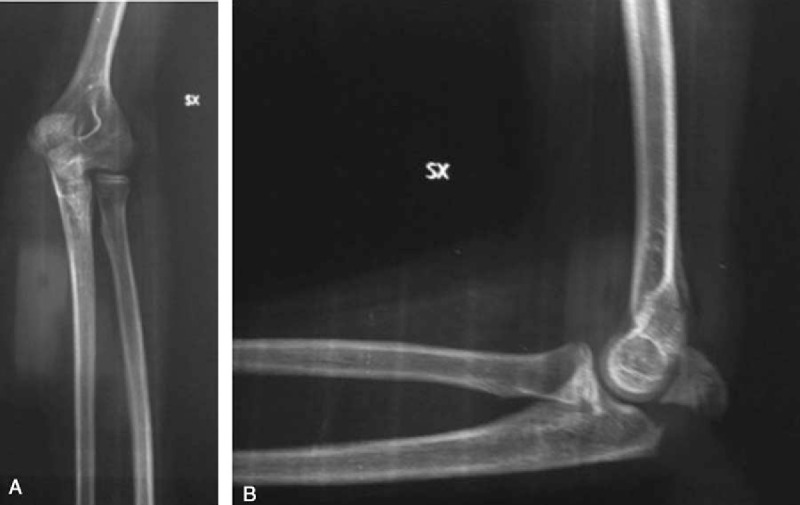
Preoperative X-rays in a male patient (12 years old), showing the fracture/avulsion of olecranon apophysis (Mayo II) in the anterior-posterior (A) and the lateral (B) planes.
